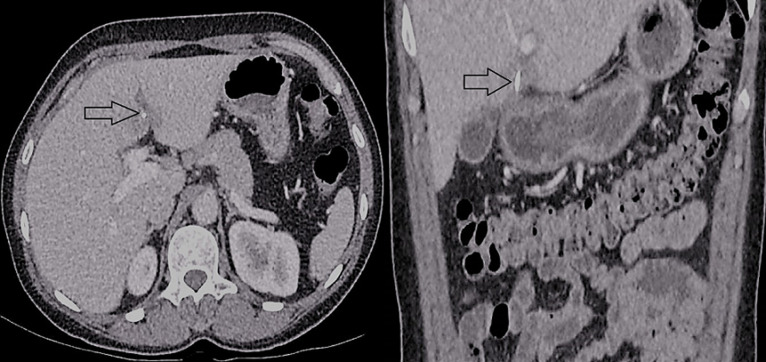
CT scan 6 weeks post presentation with resolving liver abscess and fishbone remains in place as indicated by arrows
